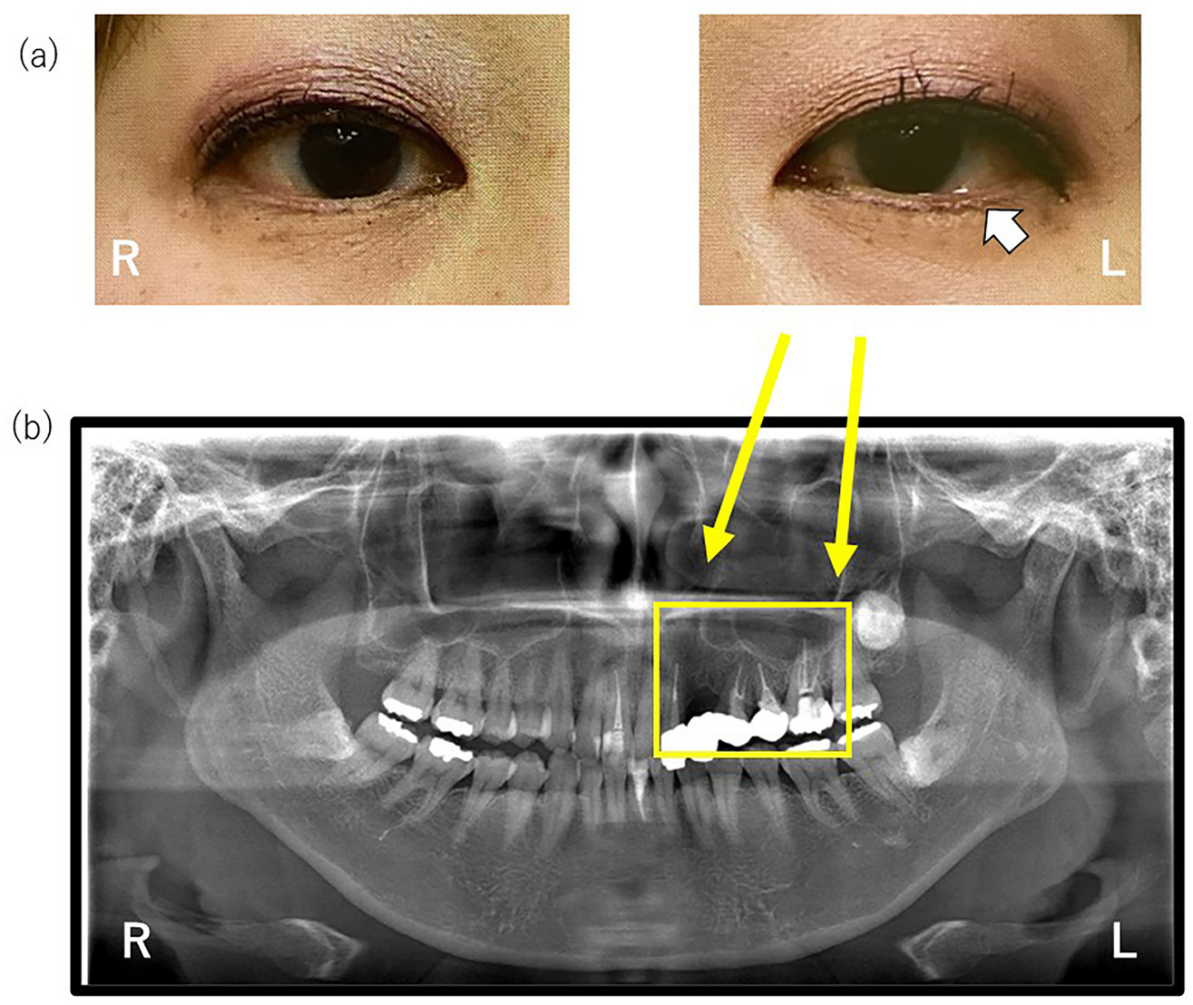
(a) Left ptosis with conjunctival injection was observed. (b) The panoramic radiographs show evidence of tooth extraction and root canal treatment predominantly in the upper left maxillary region. Right (R), Left(L).
